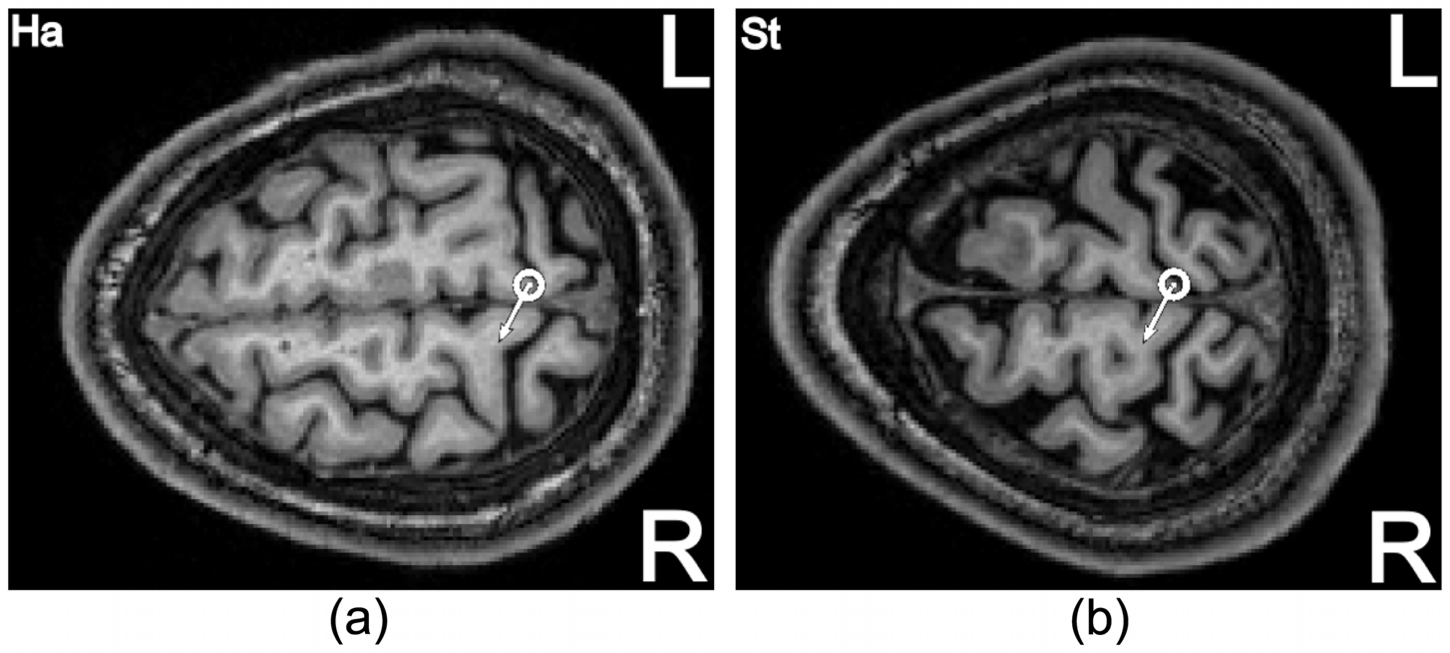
Hot-spot (dot with surrounding circle) for two subjects projected in the MRI images in a transversal view. An area of the precentral gyrus at the edge to the central sulcus and close to the interhemispheric cleft is in focus for stimulation. The white arrows denote the found optimal coil orientation angle for the individual subject.
